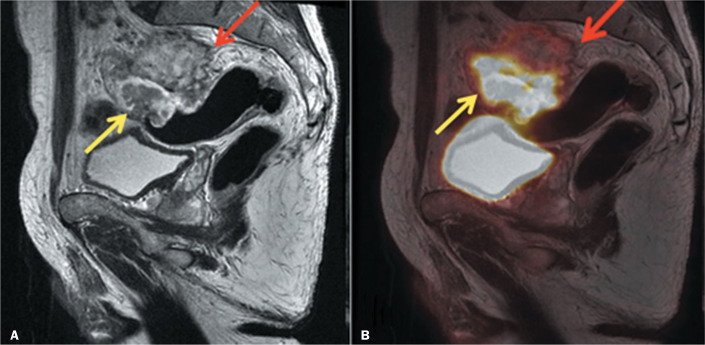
A 56-year-old male at primary staging of rectal cancer. A: MRI
